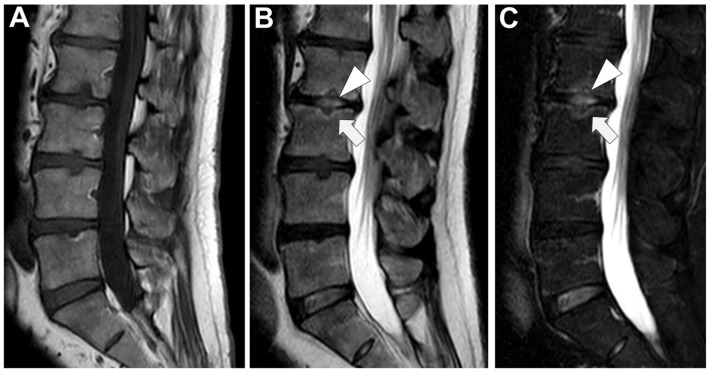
A 56-year-old male presented with a chronic and mechanic lumbar pain. An MRI was performed with (A) T1-weighted imaging (WI), (B) T2-WI, and (C) fat suppressed T2-WI. It demonstrates multiple Schmorl nodes (arrowheads) and a linear high signal intensity (SI) of the subchondral bones on both side of the L2-L3 level (arrows). There was no erosion, small anterior osteophyte, and degenerative disks. Hence, Modic 1 was diagnosed.
